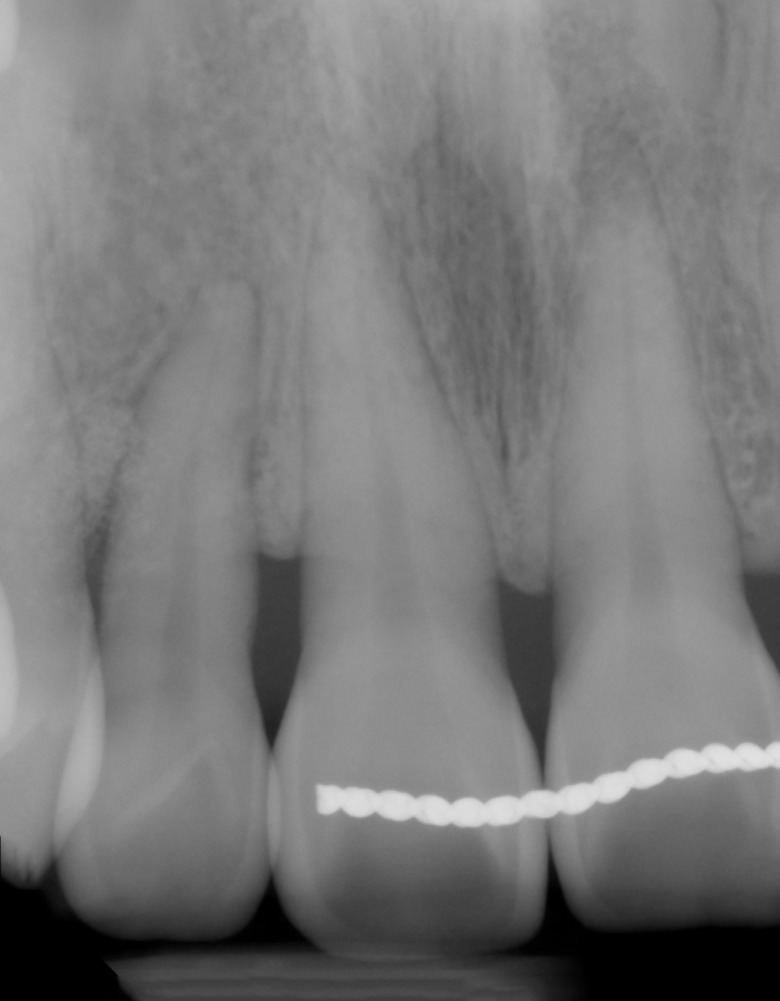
Pre-operaive and 6-month post-surgery radiographs demonstrating significant bone fill at site (tooth no. 12) in the same case as Fig 3.
